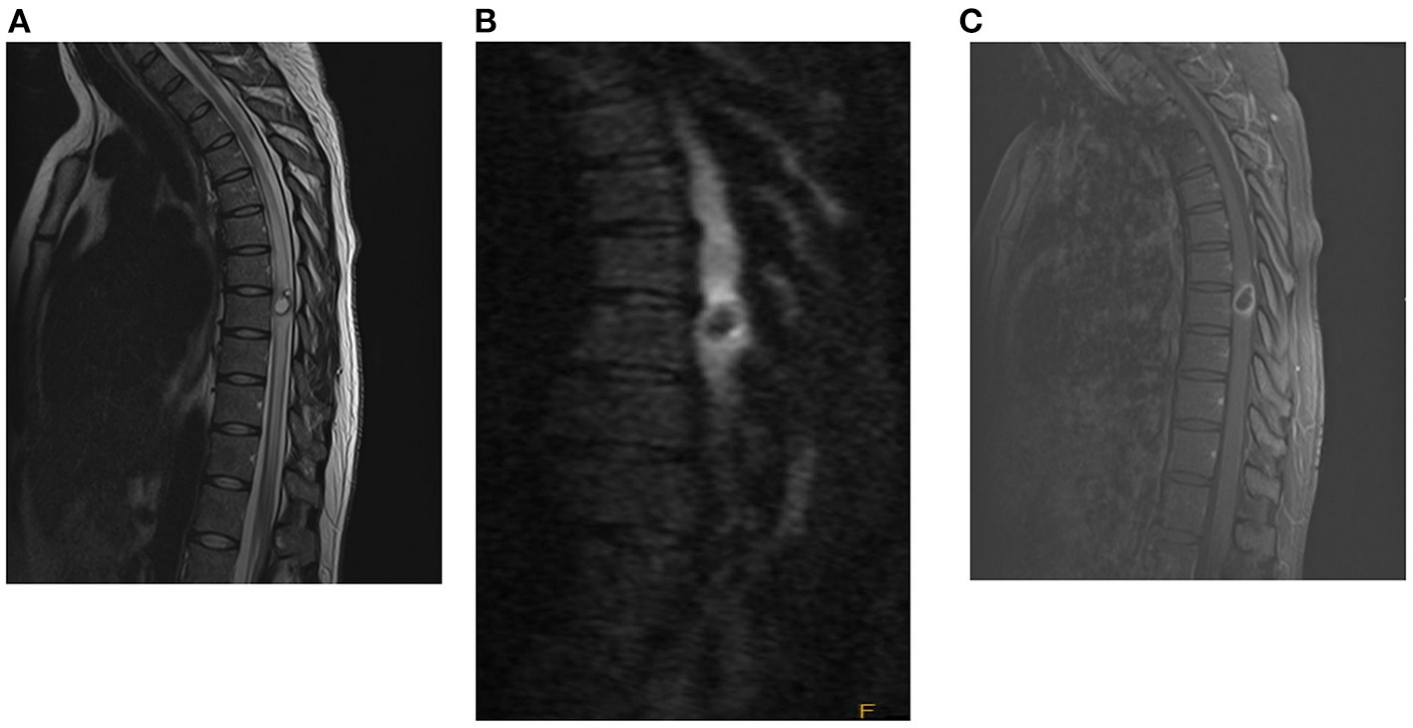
Pre-operative MRI with and without contrast of spine [(A): T2, (B): T1 wo contrast, (C): T1 w contrast], demonstrating a rim-enhancing cystic lesion in the mid-thoracic spine with diffuse surrounding cord edema.
